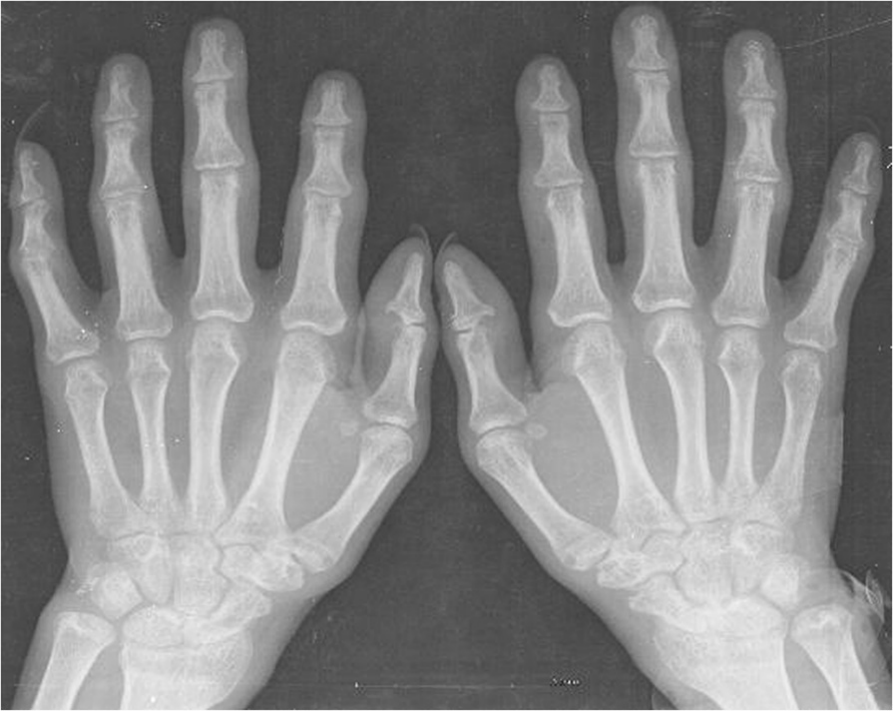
X-ray examination. This shows osteoporosis at both ends of the two hands, irregular bone destruction on part of the proximal interphalangeal joint, narrowing of local joint spaces, small fibrocystic change on part of the carpal bones and the first metacarpal base of both hands and light soft-tissue swelling.
